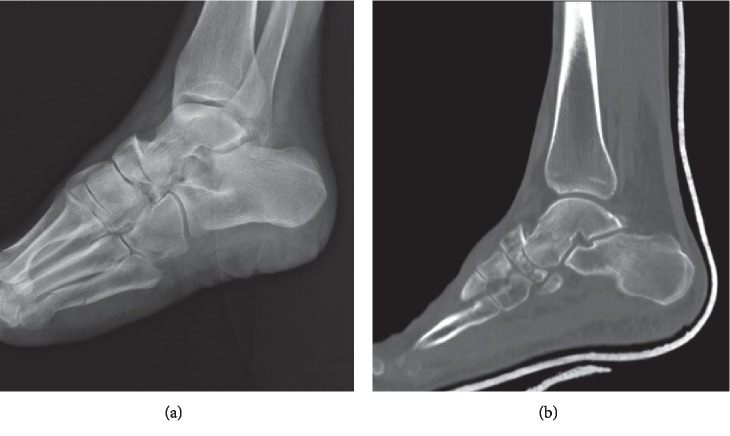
Lateral foot X-ray (a) demonstrating talonavicular fracture dislocation. In addition, there are fractures of the 4th and 5th metatarsal shafts. CT scan (b) better elucidates the fracture pattern. The talus was driven into the lateral aspect of the navicular producing a comminuted fracture.
